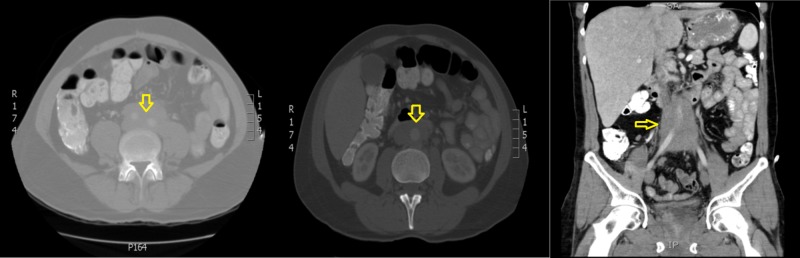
Computed tomography of the abdomen Retroperitoneal mass of infrarenal location, compromising the para-aortic, interaortocaval space in front of the aorta, extending to the aortoiliac bifurcation, with well-defined contours, surrounding vascular structures without infiltrating them, 99 x 38 x 55 mm, and associated with left hydronephrosis
